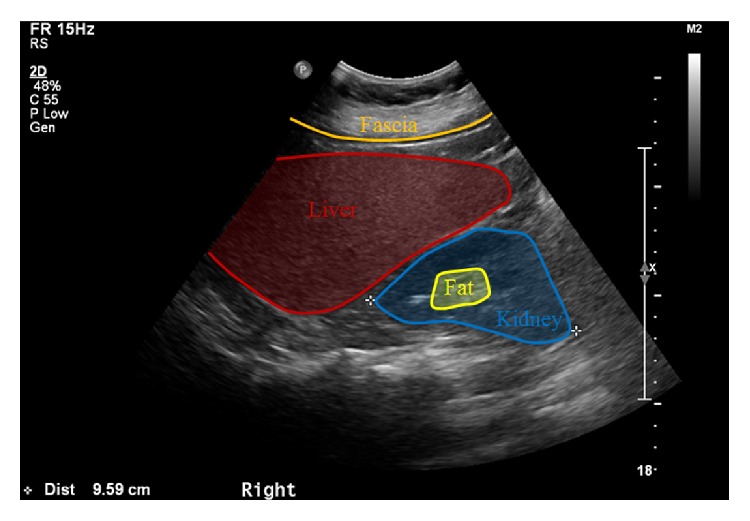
Ultrasonography image and regions of interest.
